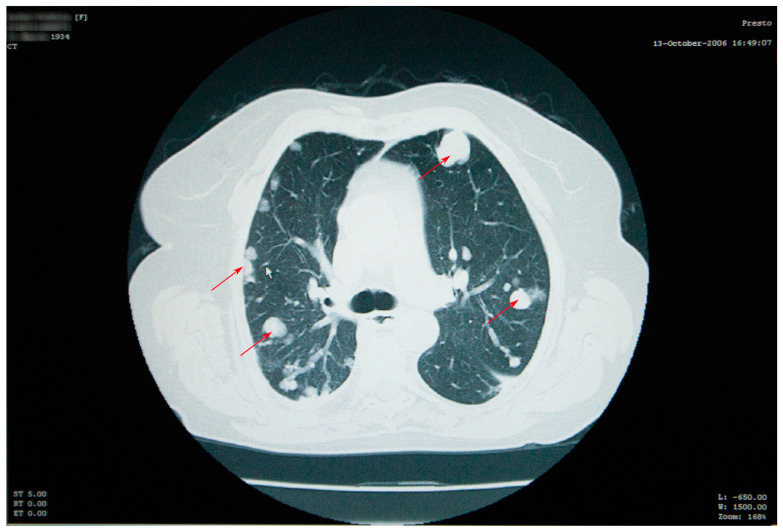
Thoracic CT scan showing numerous thoracic masses (red arrows), located bilaterally, with different consistencies, and bilateral pachypleuritis.
